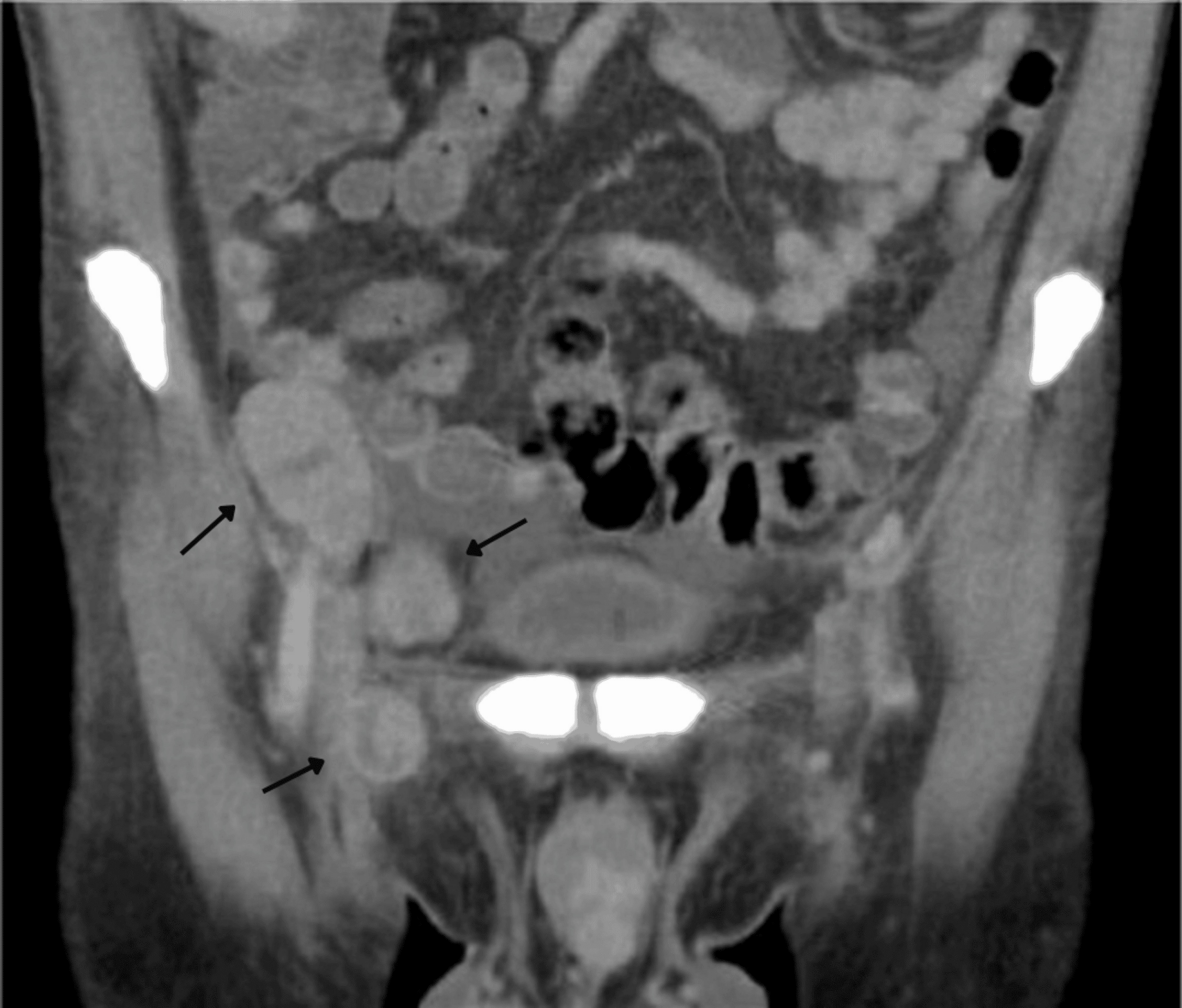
Contrast-enhanced CT of the pelvis, coronal plane. Coronal CT image demonstrates multiple enlarged bilateral inguinal and iliac lymph nodes with rounded morphology, consistent with metastatic lymphadenopathy in the context of advanced malignant melanoma. Arrows highlight metastatic lymphadenopathy. CT: computed tomography
